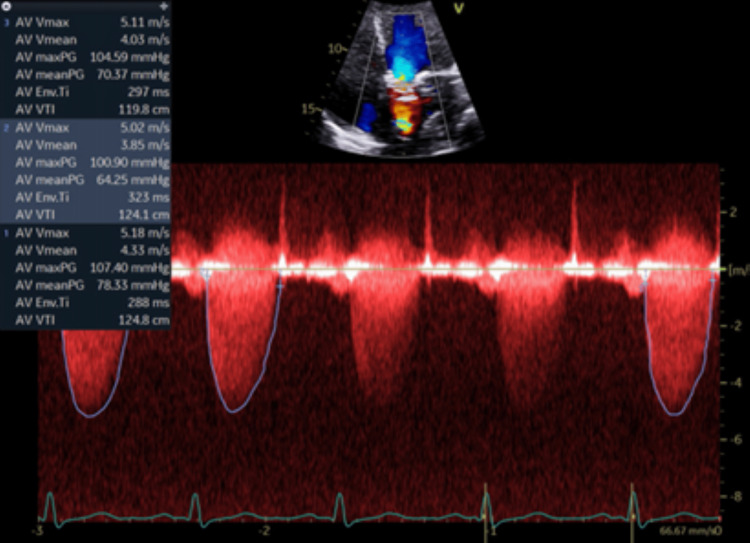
Eight Weeks Post TAVR. Thrombosed Valve while on Apixaban Color-flow doppler and velocity mapping of aortic valve showing increased transvalvular velocity with peak velocity 5.18m/s, mean gradient 78mmHg, and peak gradient 107mmHg. TAVR: transcatheter aortic valve replacement; AV: aortic valve
